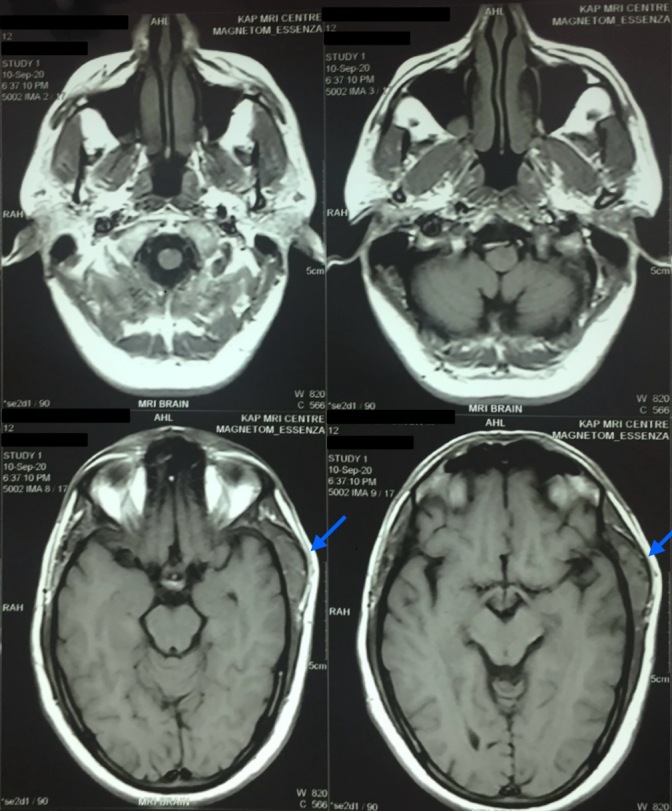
A 4.3 x 3.3 x 1.3 cm heterogenous lesion in the temporalis muscle, hyperintense on T2 weighted brain and neck MRI (axial view, indicated with blue arrows).
